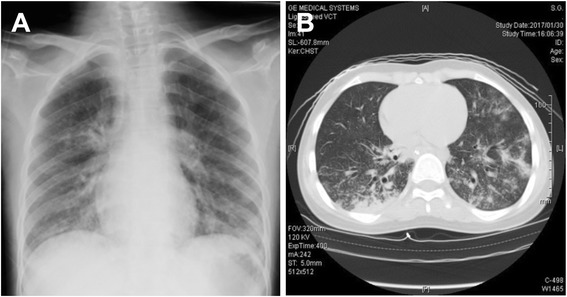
Chest presentation. a Chest X-ray on admission, showing pneumonia. b Chest CT scan on admission showing consolidation with the air bronchogram
